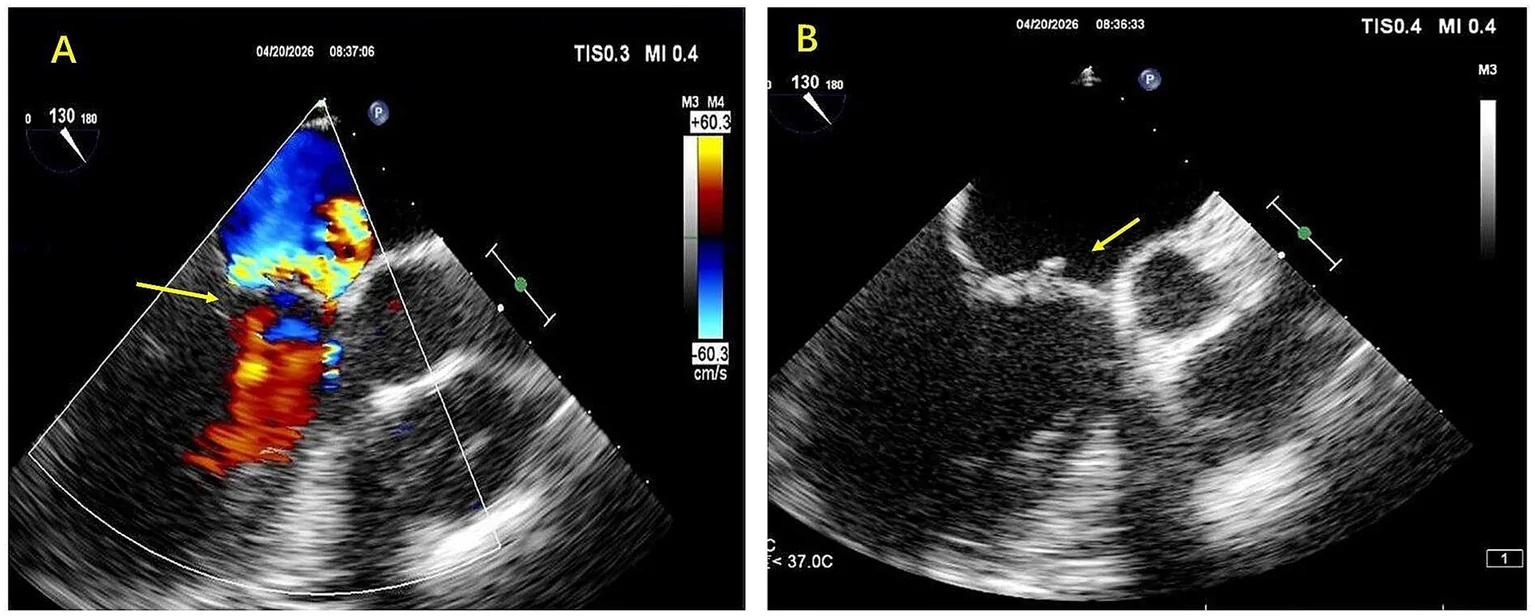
Transesophageal echocardiographic findings of the mitral valve. (A) Color Doppler transesophageal echocardiography demonstrates a mitral regurgitant jet directed into the left atrium (arrow). (B) Two-dimensional transesophageal echocardiography demonstrates a small echogenic nodular lesion attached to the atrial side of the mitral valve (arrow), suspicious for valvular vegetation.
